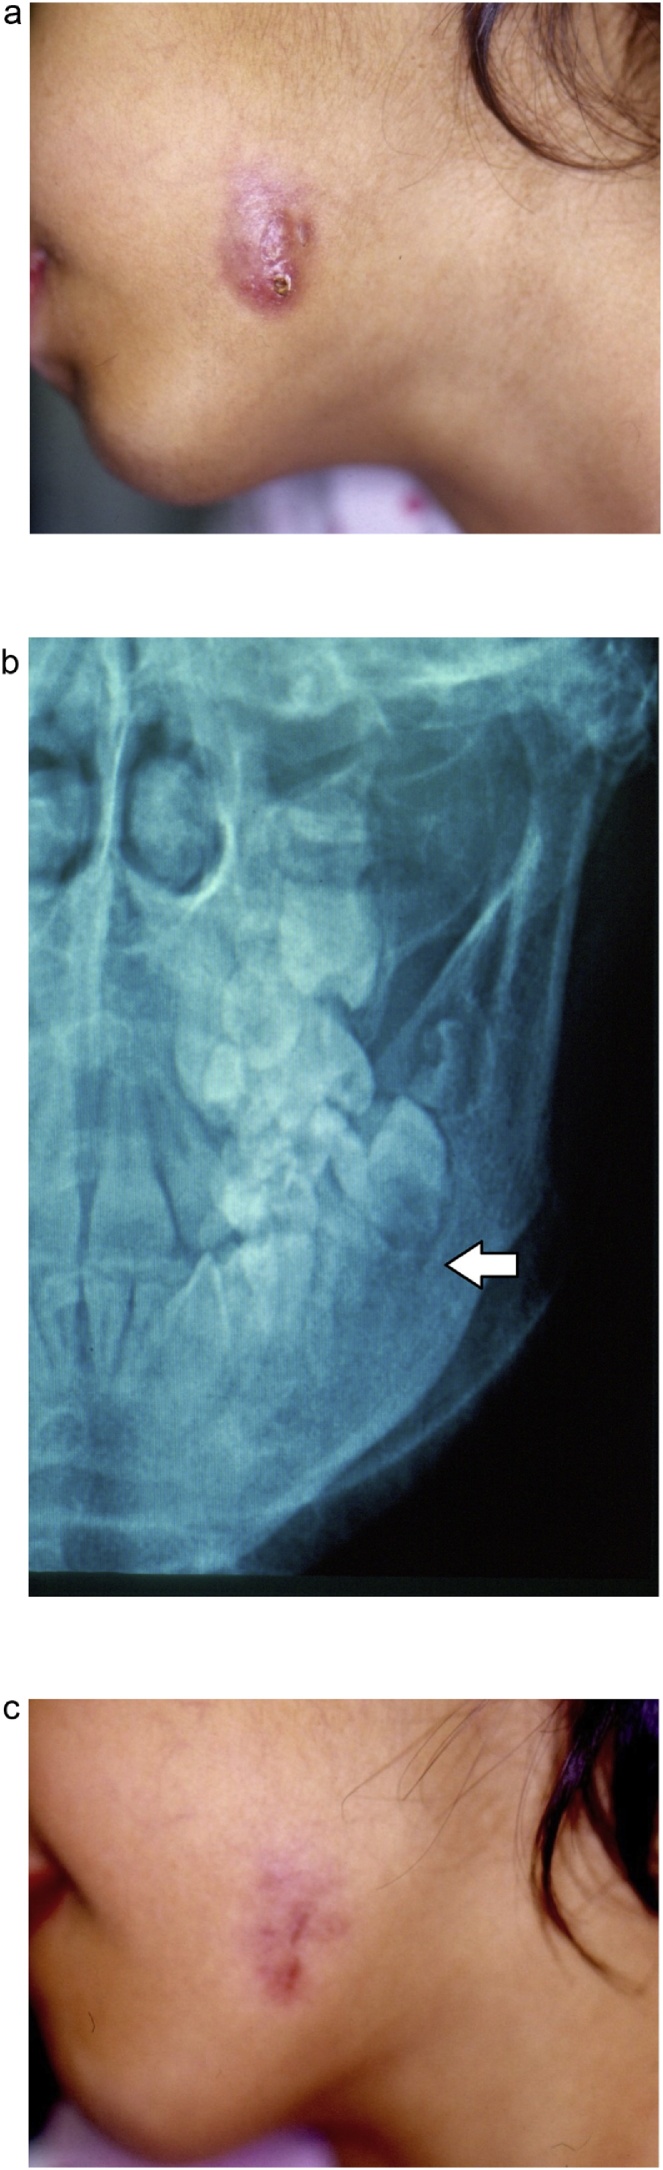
A case treated outside our clinic with excisional biopsy of the skin lesion. One month later, the patient was referred to our clinic with recurrence. a) The appearance at the time of presentation to our clinic. There is recurrence with chronic inflammation at the site of the scar. b) X-ray showing the radiolucency of the adjacent molar tooth (arrow). c) The resolution of the chronic infection after dental treatment. The remaining scar was long and was considered “unnecessary”, resulting in a medico-legal claim.
